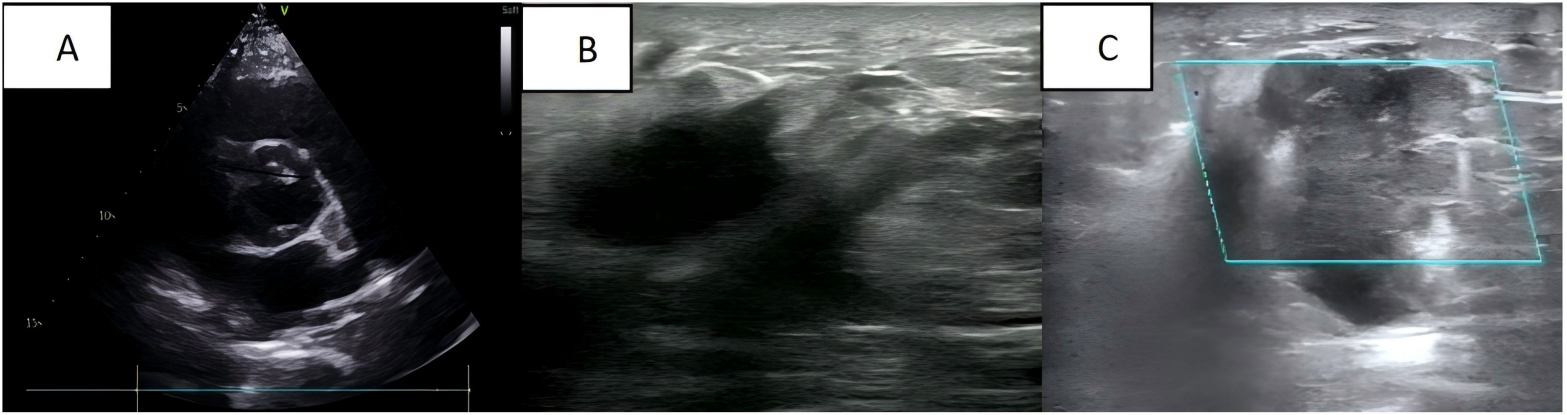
(A) Cardiac ultrasound: congenital heart changes are seen; (B) Breast ultrasound: a mass is seen in the right breast; (C) Axillary ultrasound: an enlarged lymph node is seen in the right axilla.
